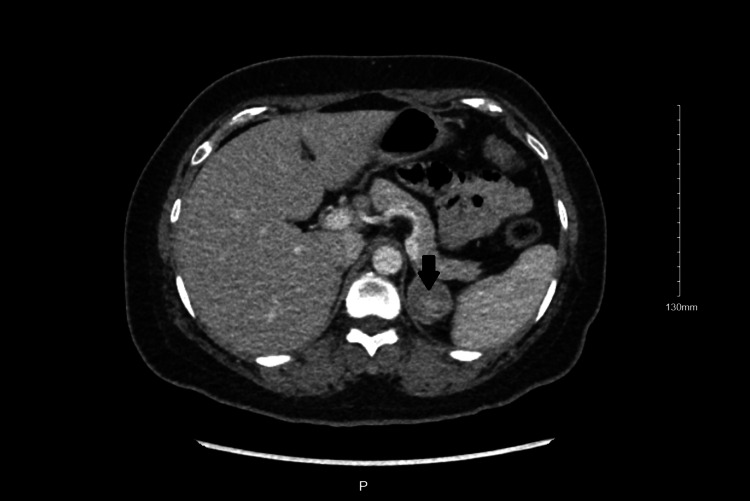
CT abdomen pelvis (transverse view) showing left-sided adrenal adenoma
